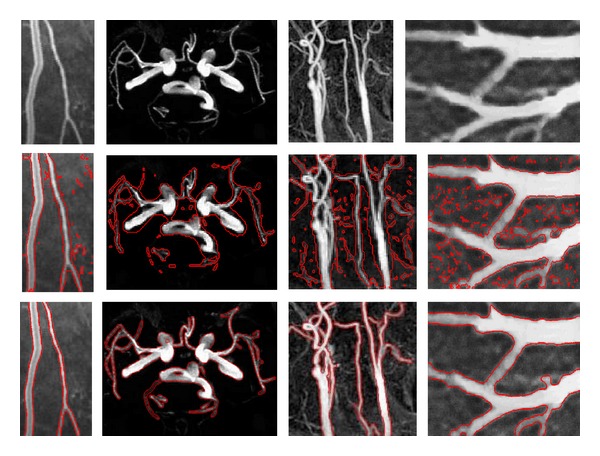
Results of the proposed model for CT, MRA, and US images. Rows 1, 2, and 3 show the original images, initial contour, and final evolution results, respectively. The image results of the different methods are shown in each column.
